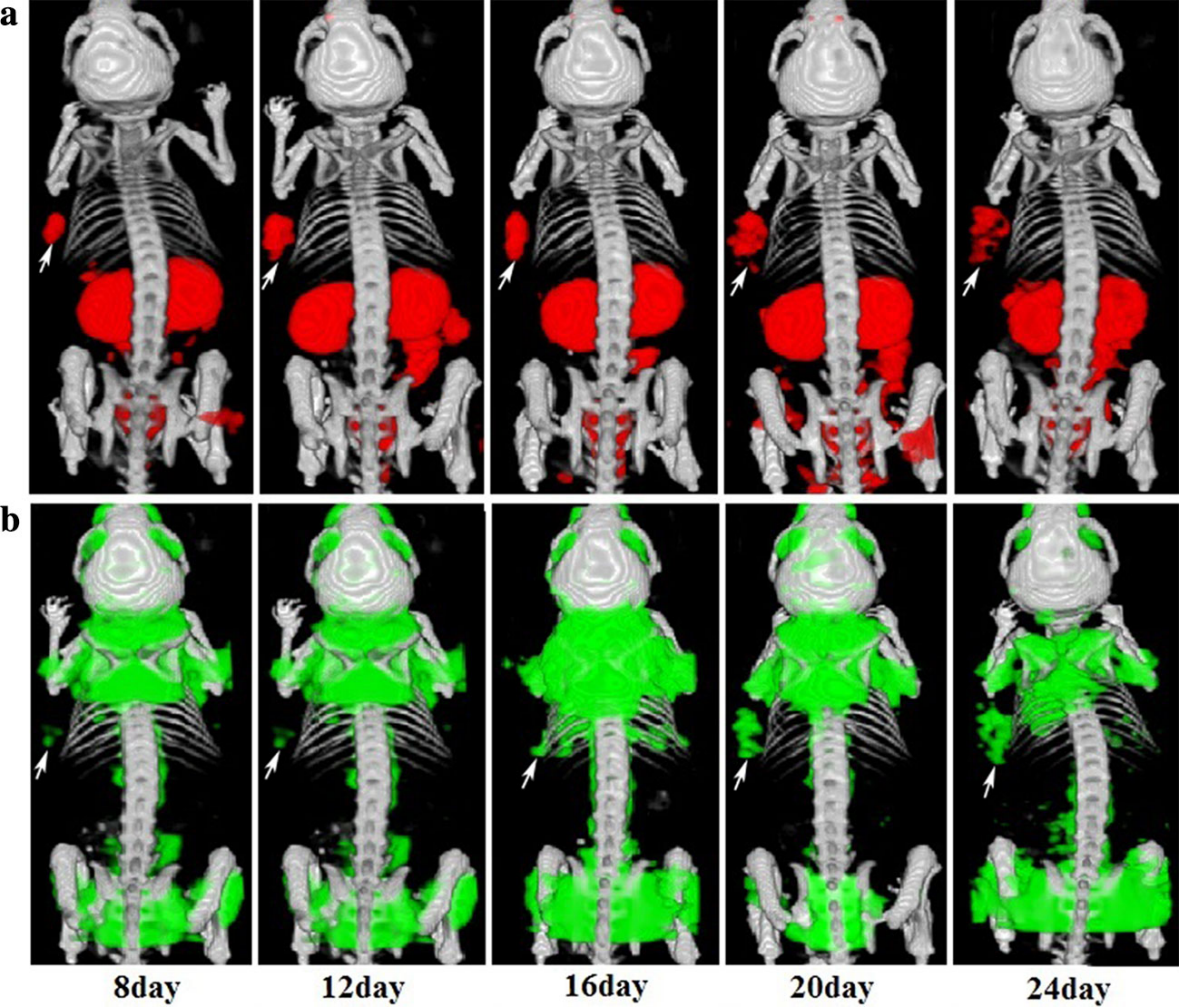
The three-dimensional views of simultaneous SPECT/PET images obtained 45 min after the injection of 55.5 MBq of ^99m^Tc-3PRGD_2_ and 37 MBq of ^18^F-FDG in ^99m^Tc-window (a) and ^18^F-widow (b), with a 45-min scanning time. These images were acquired at 8, 12, 16, 20 and 24 days after HepG2 cell inoculation
